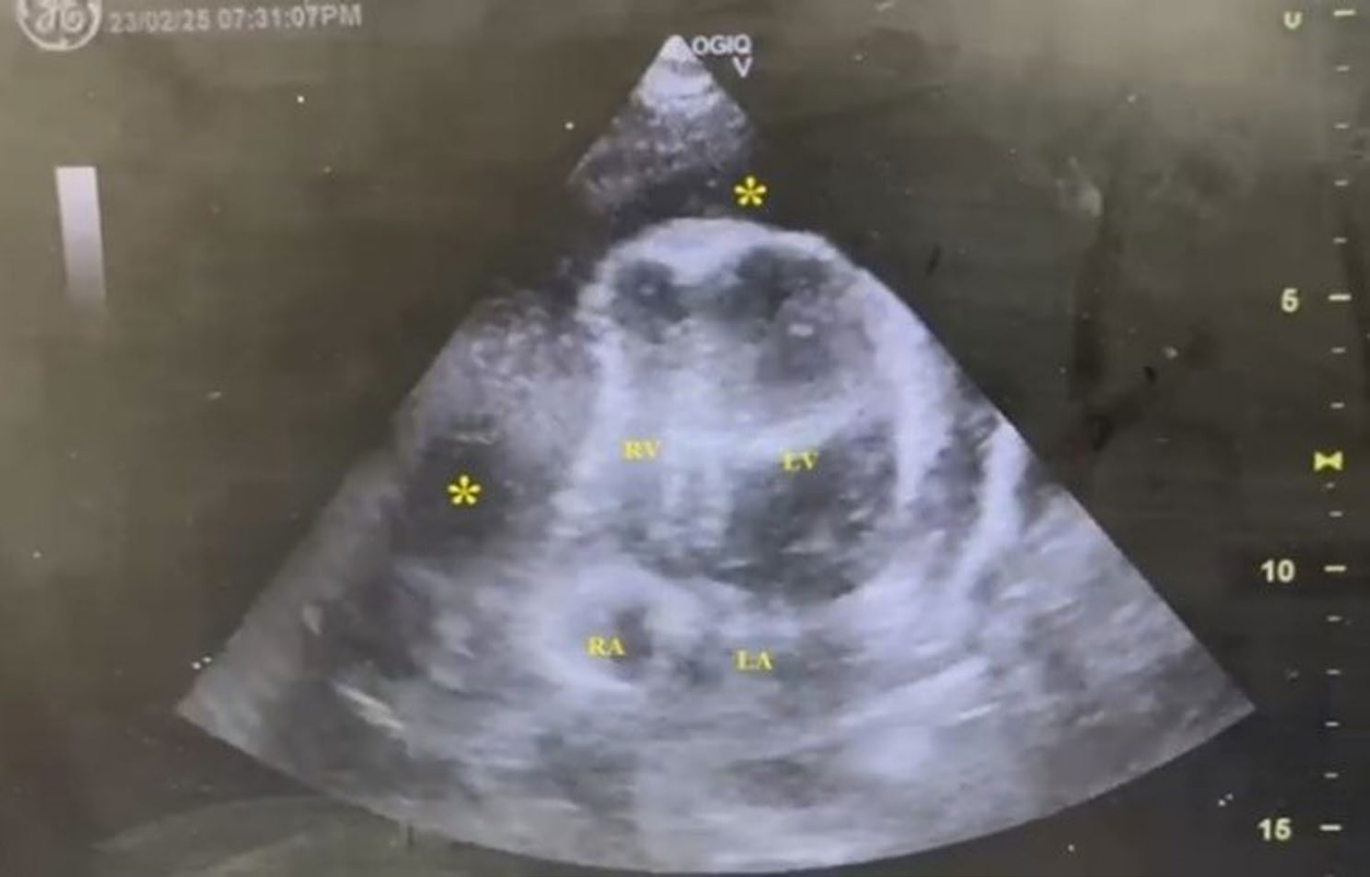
Apical 4 chamber view showing fluid in the pericardial cavity and right ventricular diastolic dysfunction. * - Pericardial effusion
